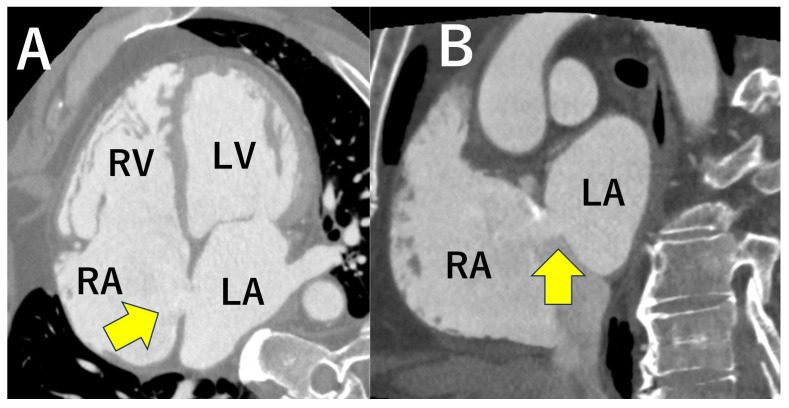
These are atrial septal defects (ASD) on computed tomography ((A): four-chamber view, (B): short axial view). The ASD secondary defect is well visualized in both images (yellow arrows). LA; Left atrium, RA; Right atrium, LV; Left ventricle, RV; Right ventricle.
